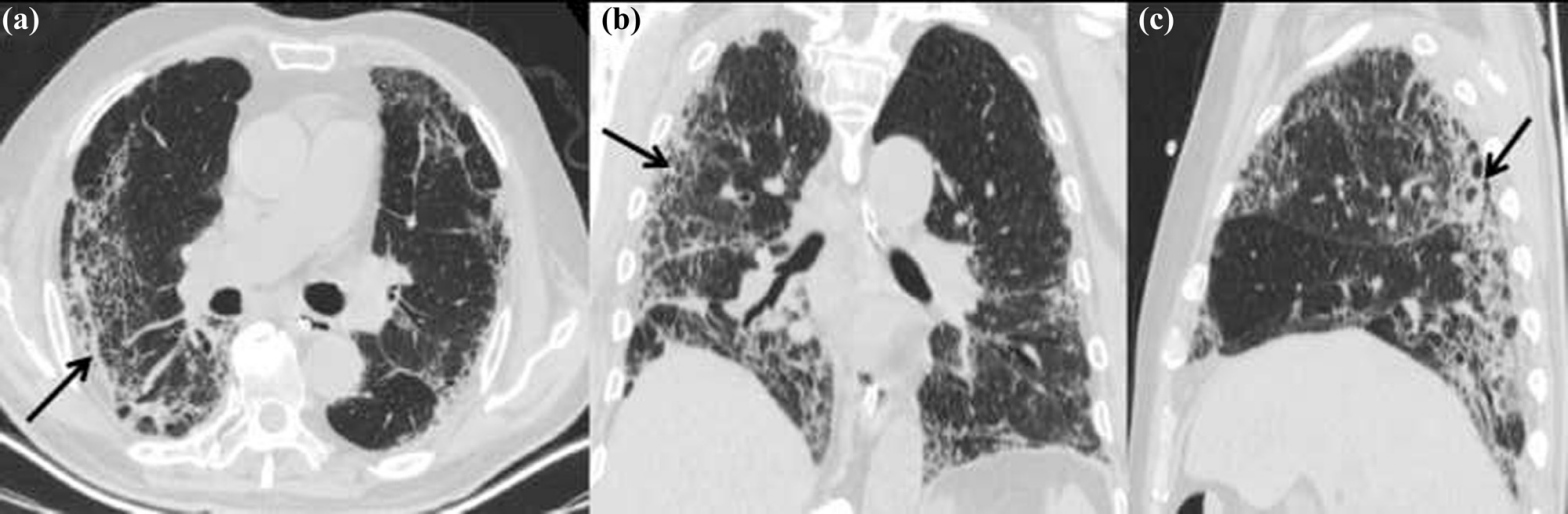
CT scans obtained 20 days after admission (a: axial non-contrast CT image; b: coronal thin-section non-contrast CT image; and c: sagittal thin-section noncontrast CT image) showing the range of lesions reduced significantly compared with previous images; fibrous changes in subpleural (black arrows).
